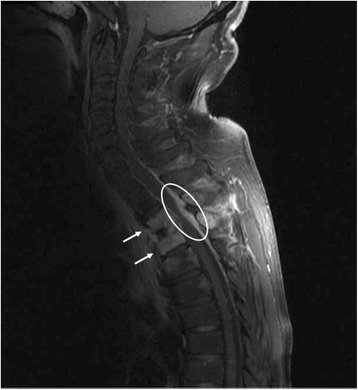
MRI at the onset of paraplegia showed the destructions of the Th2–3 vertebral bodies (arrow) and the intervertebral disc accompanying epidural abscess (circled high intensity area) compressing the spinal cord at the level of Th2–3
